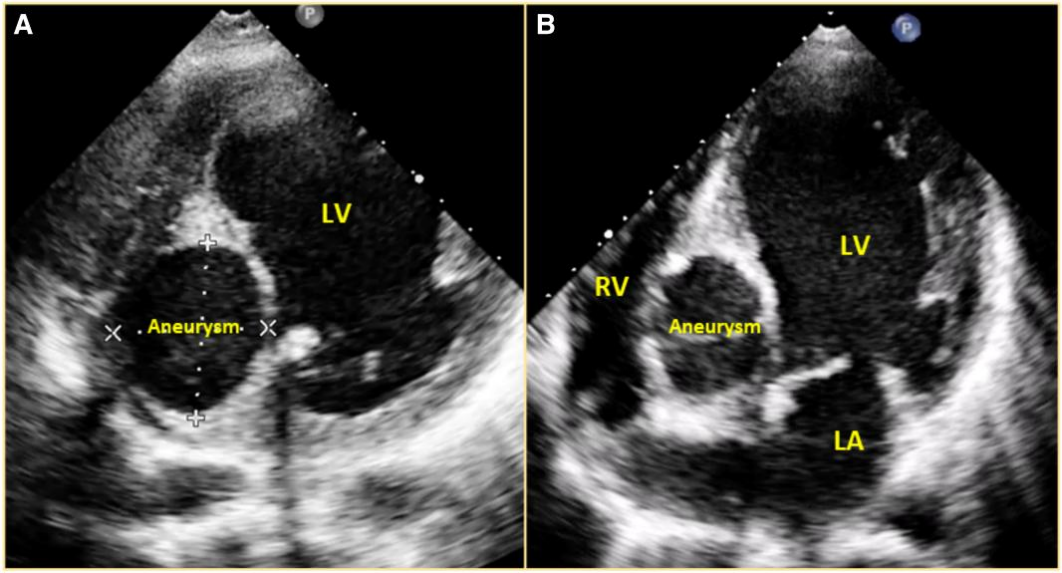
2D echocardiogram in modified short axis view in midventricular segment (*A*) and modified apical four-chamber view (*B*) showing 37 mm × 42 mm aneurysm dissecting into the interventricular septum with calcified walls. LV, left ventricle; RV, right ventricle; LA, left atrium.
